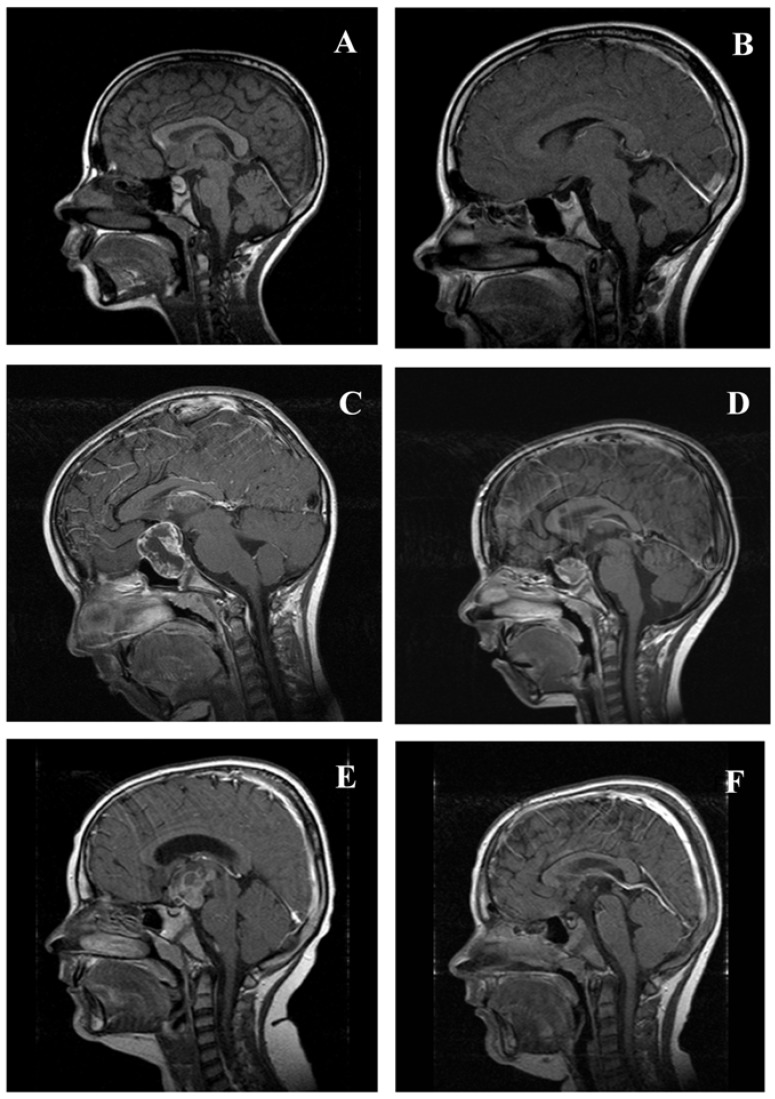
BMI and MRI imaging at diagnosis and 36 months after surgery in three cases of childhood-onset craniopharyngioma (CP) with different grades of hypothalamic involvement/lesion. (A,B) CP confined to the intrasellar space (0° no hypothalamic involvement (A)/surgical lesion (B)). BMI at diagnosis: −0.11 S.D.; BMI 36 months after complete resection: −0.41 S.D. (C,D) CP involving the anterior hypothalamus (I° hypothalamic involvement (C)/surgical lesion of the anterior hypothalamic area (D)). BMI at diagnosis: −1.75 S.D.; BMI 36 months after complete resection: −0.43 S.D. (E,F) CP involving the anterior and posterior hypothalamus (II° hypothalamic involvement (E)/surgical lesion of the anterior and posterior hypothalamic area (F)). BMI at diagnosis: +6.08 S.D.; BMI 36 months after complete resection: +6.79 S.D. Modified from [46], with kind permission from Bioscientifica.
